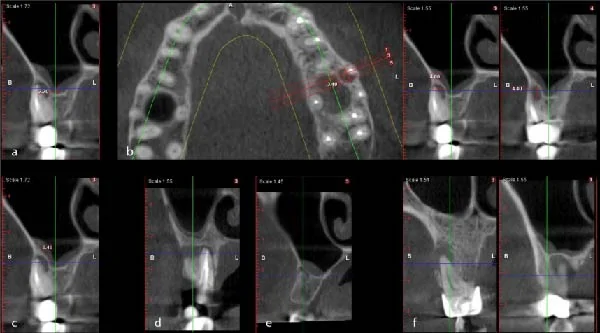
Representative CBCT images showing the radiographic variables evaluated in this study: (a) Schneiderian membrane thickness (SMT), (b) periapical lesion dimensions used for lesion volume estimation, (c) minimum bone thickness between the lesion and the sinus floor, (d) flat mucosal thickening, (e) round/curved mucosal morphology, and (f) alveolar bone plate perforation.
